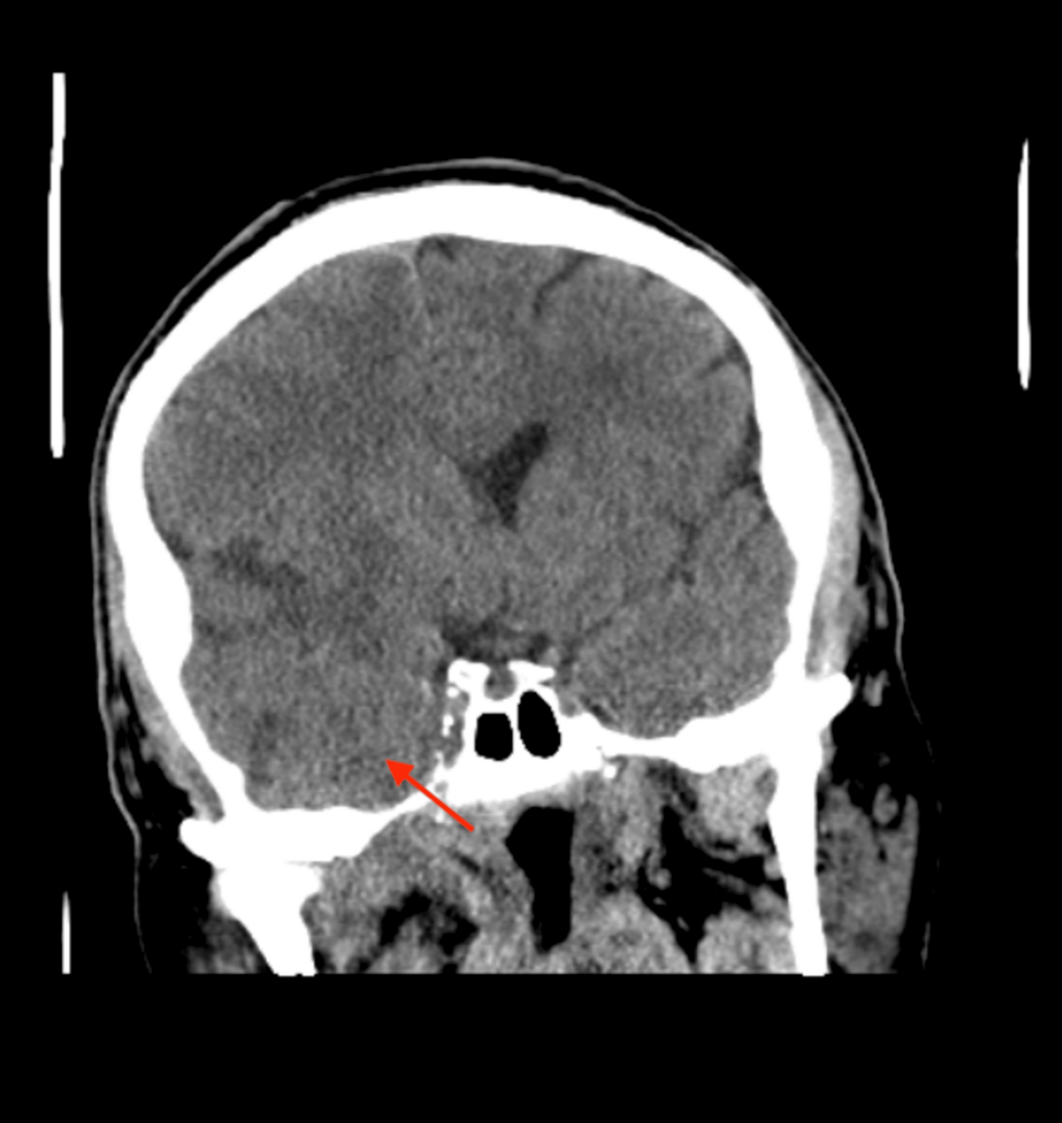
CT scan of the head (coronal section) A CT scan of the head (coronal section) showed cerebral edema in the right frontal and temporal lobes (red arrow), suggesting a possible underlying metastatic process with mass effect.
